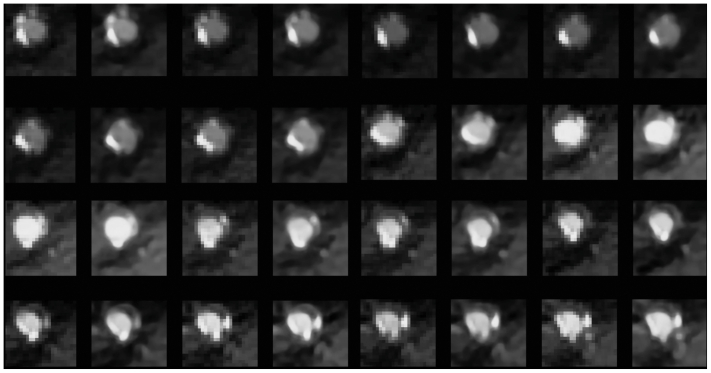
Example of a sequence of computed tomography (CT) and super-resolution (SR)-CT images. From *left* to *right*, the pairs of images [low-resolution (LR) CT, reconstructed SR-CT). The CT images correspond to a sequence of two-dimensional (2D) slices of a three-dimensional (3D) CT image, where the calcifications are present. The corresponding ST-CT images are obtained with the LapSRN algorithm with a factor of upscaling of eight.
